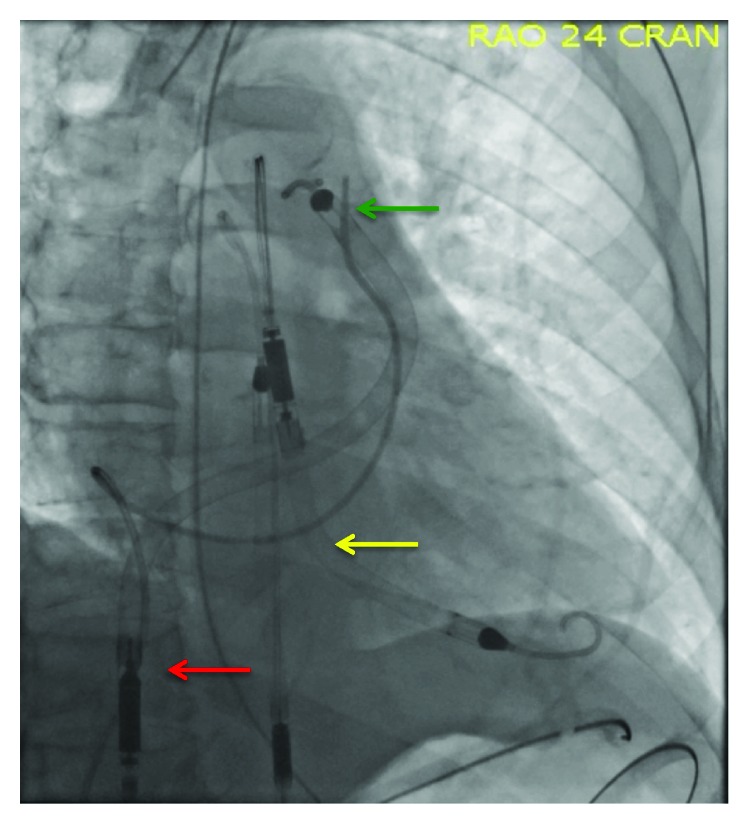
Chest X-ray showing RP Impella with the inlet situated in the inferior vena cava (red arrow) and the outlet in the pulmonary artery (green arrow) and Impella 5.0 with the inlet in the left ventricle and the outlet in the ascending aorta (yellow arrow).
